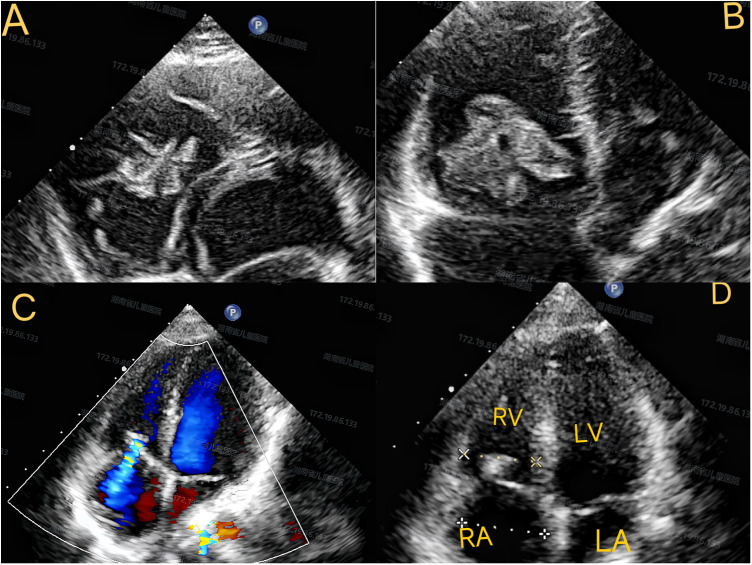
Transthoracic echocardiography images. (A) Apical four-chamber view showing a hyperechoic mass (30 × 25 × 21 mm) attached to the anterior leaflet of the tricuspid valve. (B) The mass that prolapses into the right ventricle during diastole. (C) Transesophageal echocardiography confirming complete excision. (D) Postoperative follow-up showing mild tricuspid regurgitation. RA, right atrium; RV, right ventricle; LA, left atrium; LV, left ventricle.
